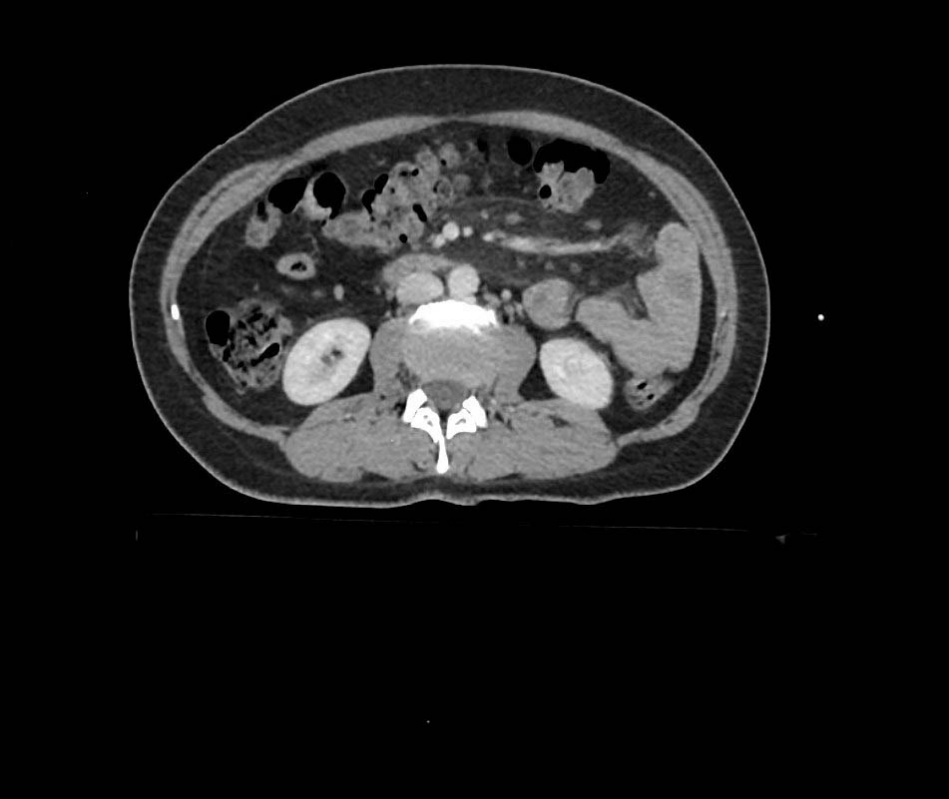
Contrast-enhanced CT scan shows a well-defined
